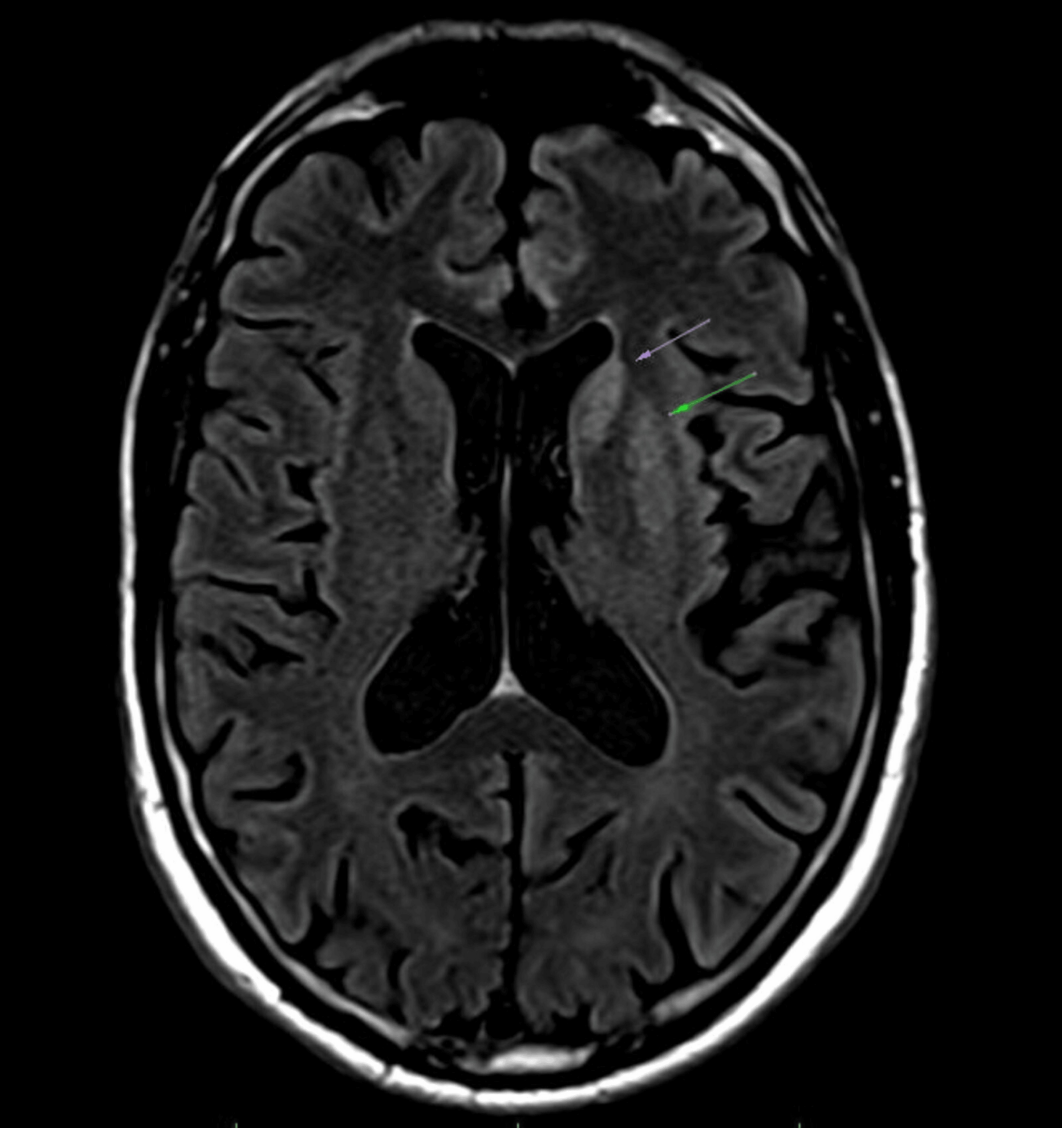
MRI showing T2 flair in the left hemisphere, precisely in the left basal ganglia, highlighted by the arrows
